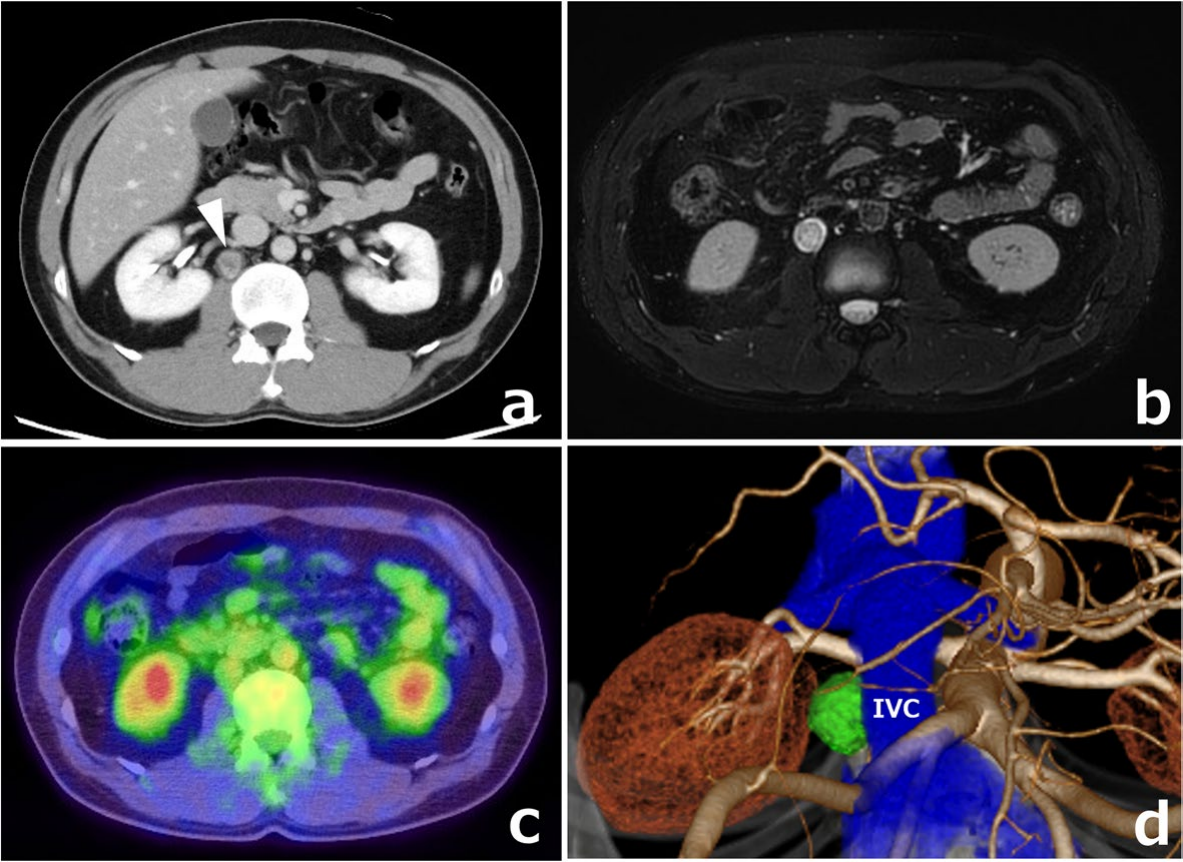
a CT showed a tumor (white arrowhead) on the dorsal side of the IVC. b T2-weighted MRI showed a high-intensity lesion. c A mass with low FDG uptake was observed on PET-CT. d SYNAPSE VINCENT was used to convert DICOM data of the CT images to 3D images
